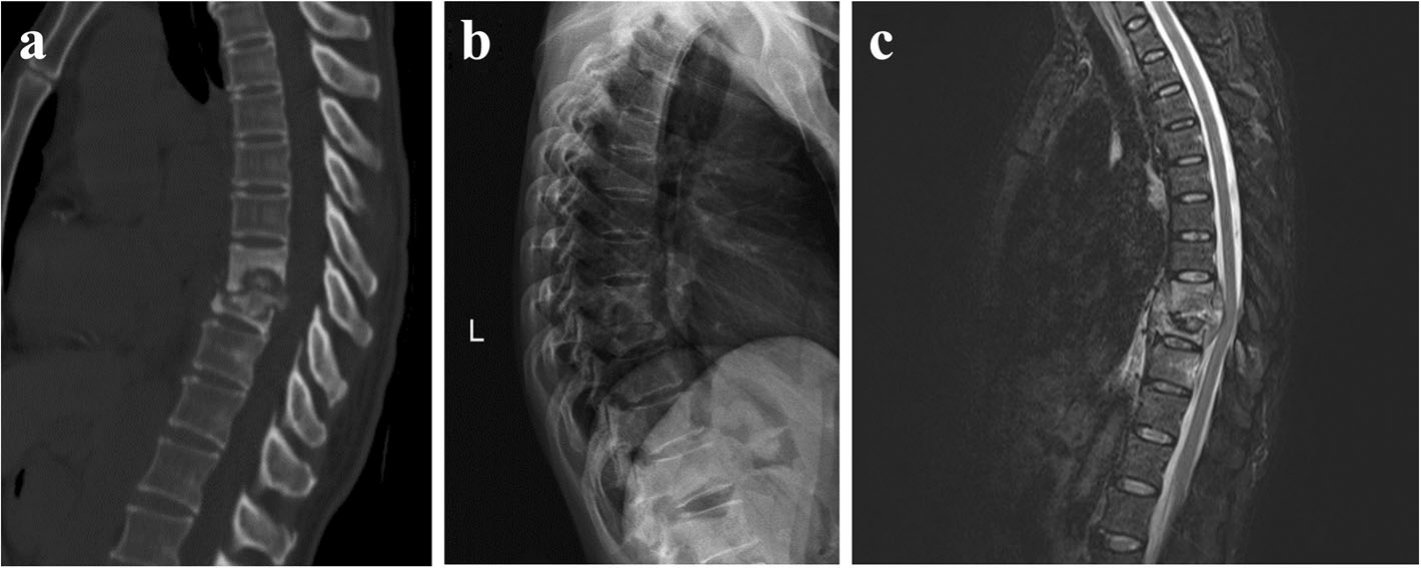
A patient with thoracic spinal tuberculosis at T7/8 received digital radiography (DR), computed tomography (CT) and magnetic resonance imaging (MRI) examinations before the operation. a Preoperative CT scan image showed bone defect at T7/8 with disc space narrowing. b Preoperative DR image. c Preoperative MRI image showed T7/8 vertebral tuberculosis with paraspinal abscess
